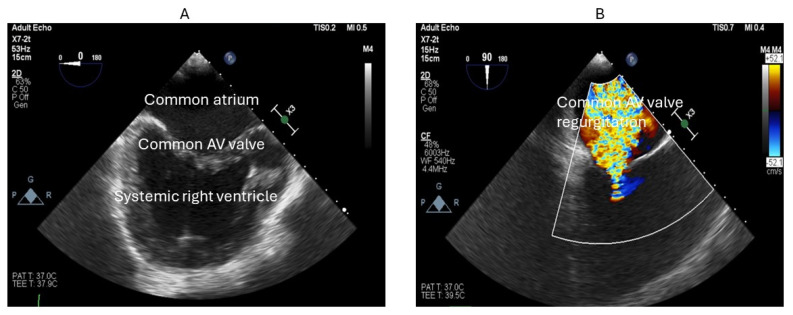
Transesophageal echocardiogram demonstrating an enlarged common atrium with a deformed atrioventricular valve (AV) (A) and severe AV valve regurgitation (B).
